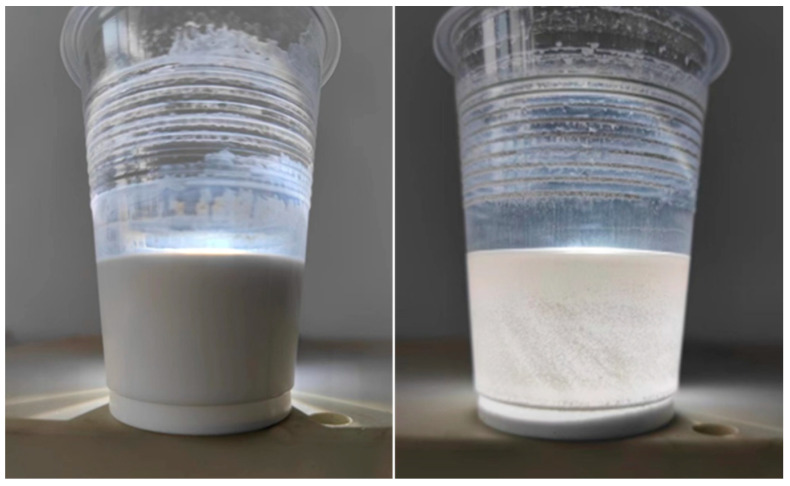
Ultrasonically dispersed (left) and magnetically stirred (right) ZnO-3 sample (after standing for 24 h).
